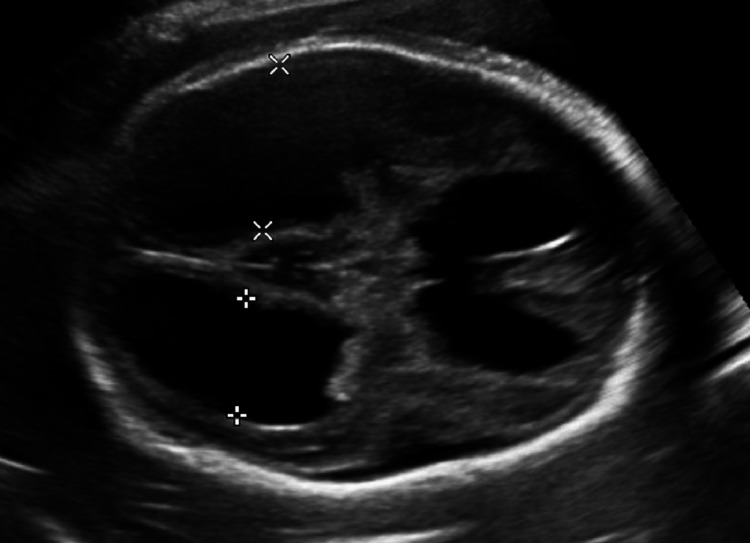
Ultrasound after the fourth transfusion showing severe ventriculomegaly (24 mm)
